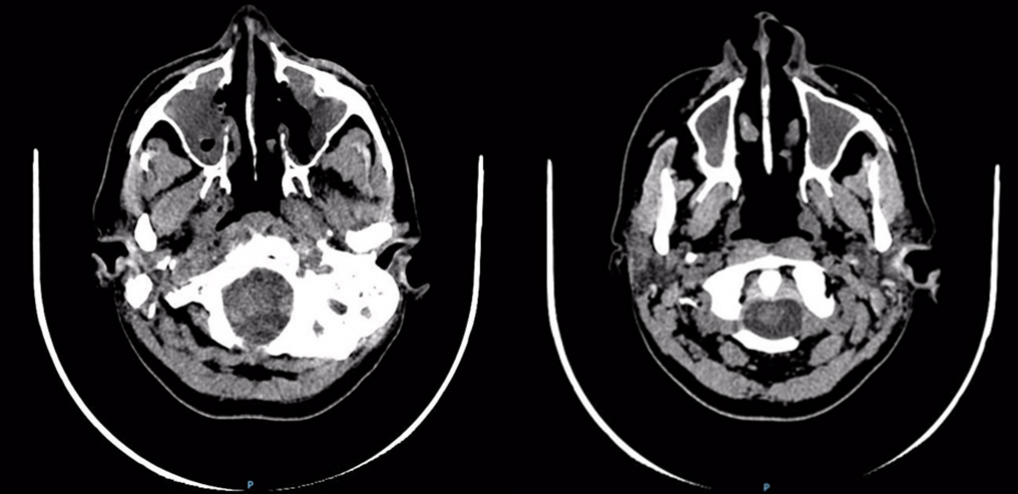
Cranial computed tomography in axial view showing an extensive compromise
